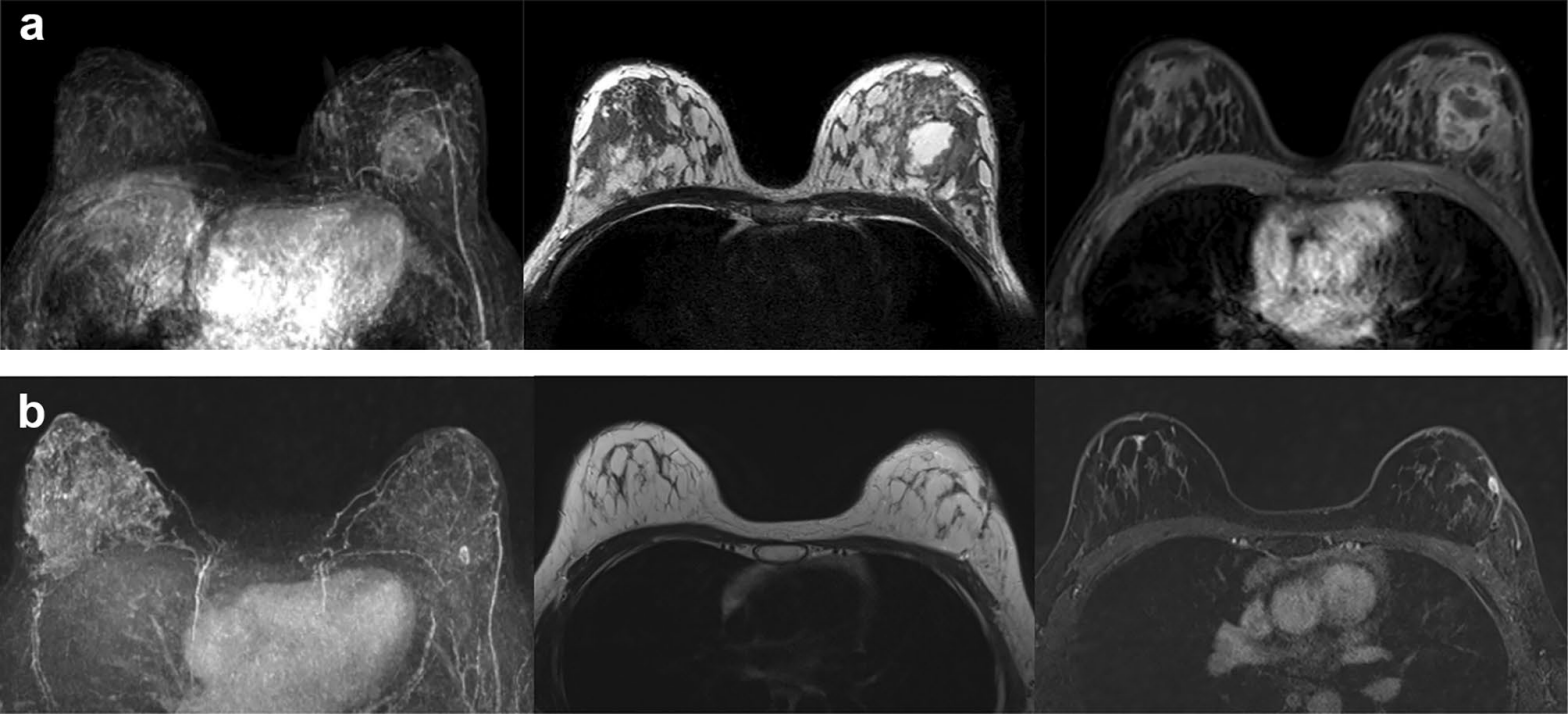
MRI of a 33-year-old female patient with left breast cancer (invasive ductal carcinoma, triple negative subtype). (a) Pre-treatment DCE-MRI. On MIP image, moderate BPE of both breasts and increased vascularity around the mass are noted. On non-fat saturated T2WI, mass shows internal necrotic change with underlying heterogenous fibroglandular tissue. On early phase of contrast enhanced fat saturated T1WI, mass shows oval circumscribed margin with internal heterogenous enhancement at 2 o’clock direction of left breast. (b) Post-operative DCE-MRI 12 months after surgery. On MIP image, a small circumscribed brightly enhancing nodule is noted at left breast. On non-fat saturated T2WI, an oval circumscribed nodule with low to intermediate signal intensity is noted near the previous excision site. This nodule shows rim enhancement pattern on early phase of contrast enhanced fat saturated T1WI. This lesion was confirmed as recurrent breast cancer (IDC, triple negative subtype) by US-guided core needle biopsy.
